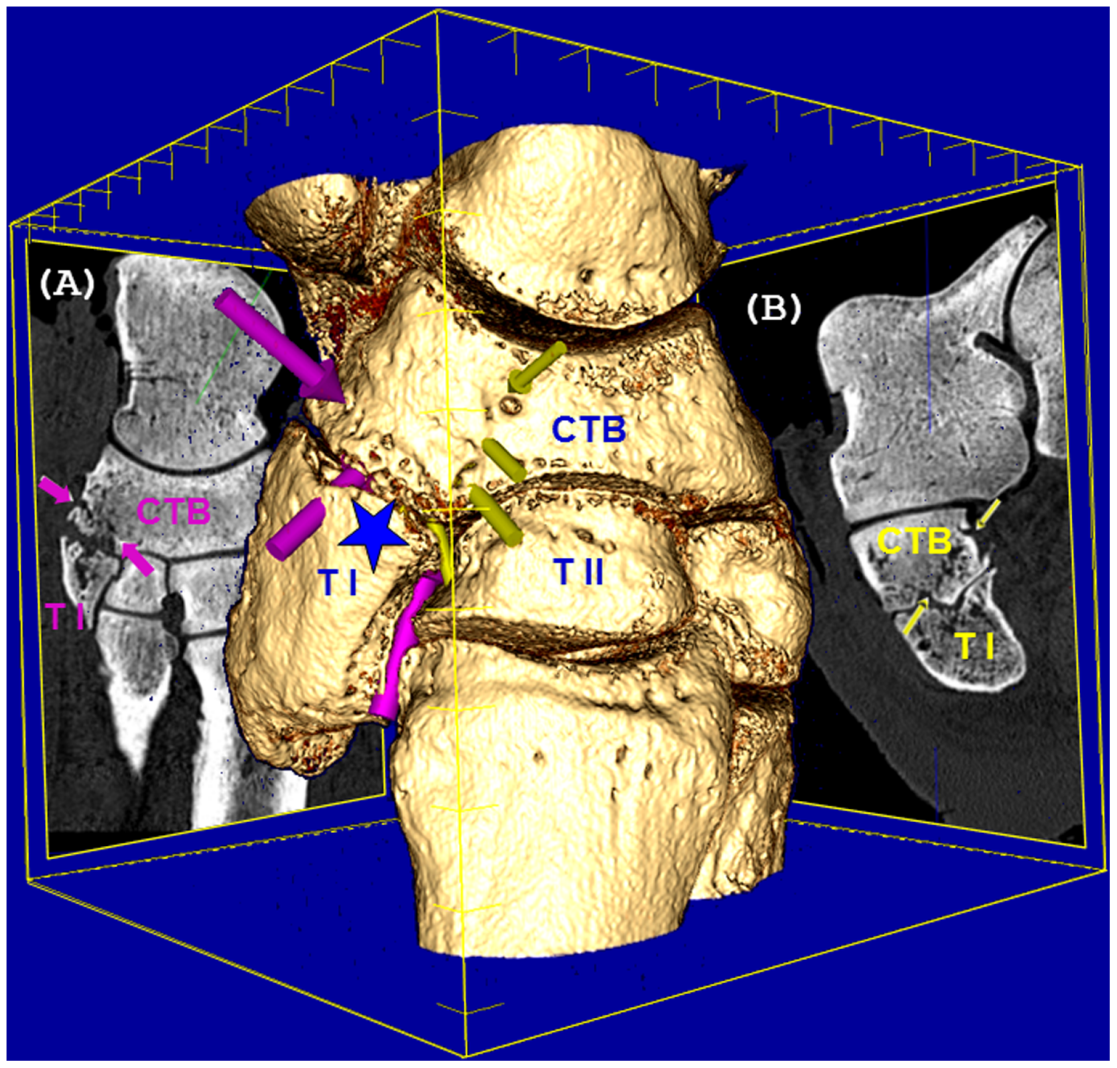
Left central tarsal bone (CTB) fractures in rhinoceros 1. Tridimensional computed tomographic reconstruction of the left tarsal joint with oblique multiplanar reconstructions (MPR) showing minimally displaced, multiple fractures of the left CTB situated in different planes (arrows). First line of fracture (A) descends in proximo-plantar to distalo-dorsal direction (large arrows), ending at the junction between CTB, first tarsal bone (T I) and second tarsal bone (T II). The second line of fracture (B) is oriented from dorsal to plantar surfaces, in proximo-medial to distalo-plantar direction, reaching the midline of the proximal articular surface of T I with CTB (small arrows). At the level of these fractures, CTB distalo-medial aspect reveals a mixed pattern of trabecular focal bone loss (osteolysis) and cortical osteogenesis represented by massive, unstructured new bone production and remodelling, with a beak-like formation oriented plantaro-medially, hook-shaped in axial plane. Additionally, the articular surface between CTB and TI is highly irregular, characterized by decreased joint space width, articular bone proliferation that bridges the contiguous bones (ankylosis), erosion and lysis of the articular cartilage and underlying bone (asterisk).
